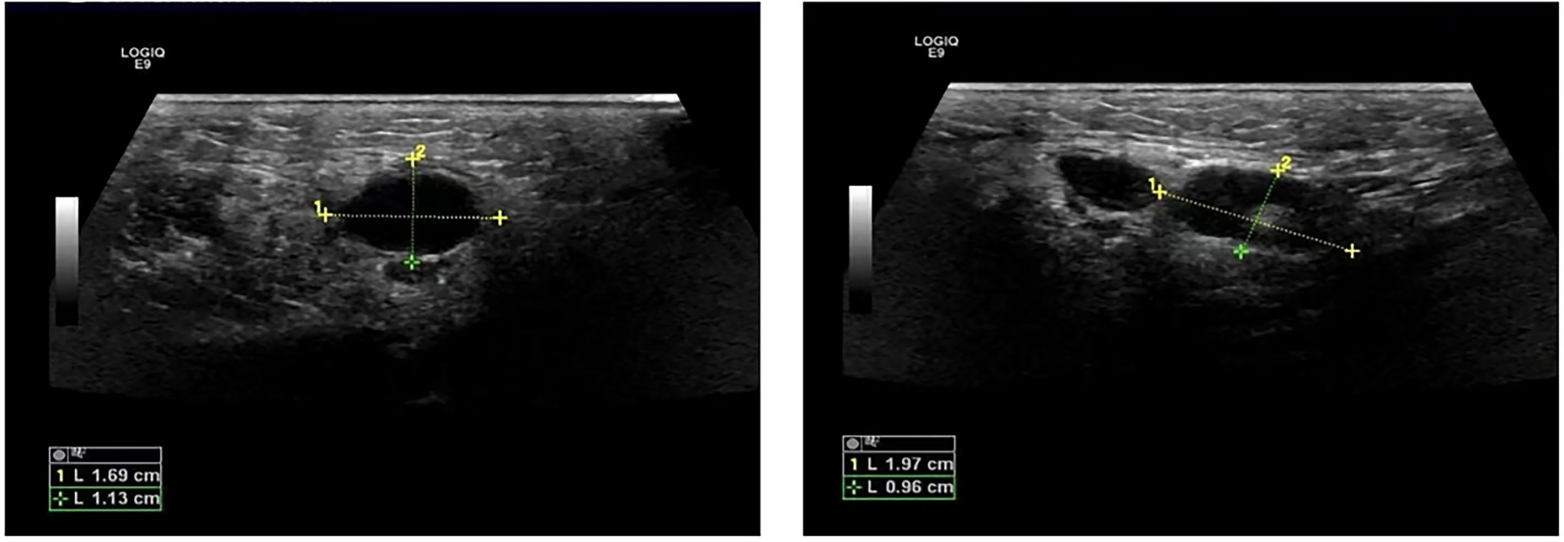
Ultrasound images of abscess.
